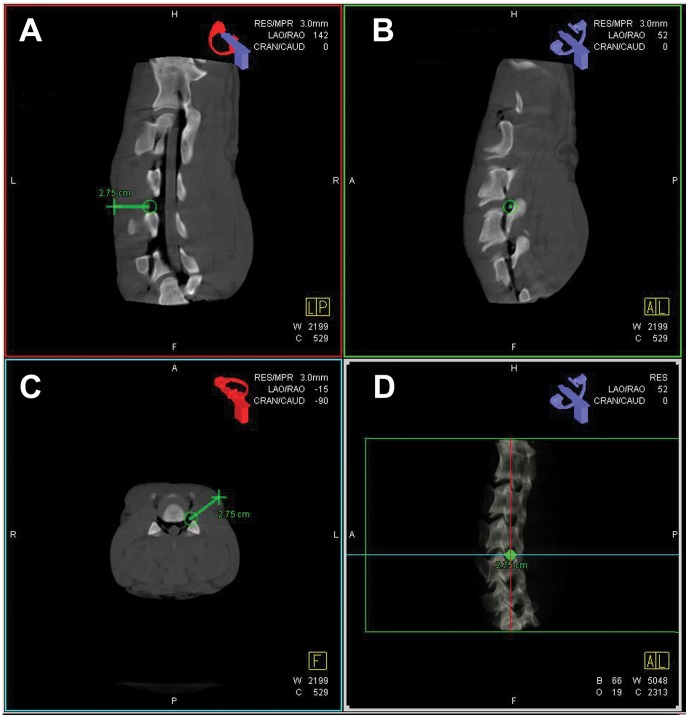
Three-dimensional puncture planning using syngo iGuide®. Three-dimensional puncture planning using syngo iGuide® with indication of the planned needle trajectory in two angulated lateral (A and B) views and the axial (C) orientation on the multiplanar Dyna-CT reconstructions of the *ex-vivo* lamb spine-model. Additionally three-dimensional rendering of the Dyna-CT (D) visualizing the trajectory path. The distance between skin entry and target area is indicated (A, C, and D).
